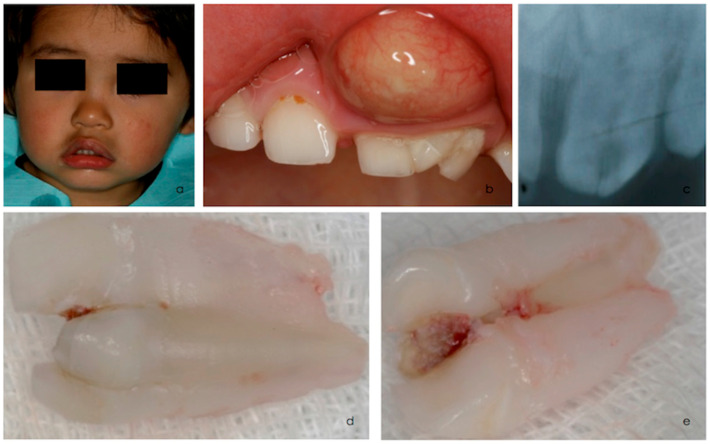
(a–c). Extraoral and intraoral exam. Intraoral periapical radiograph shows fusion of two primary incisors with supernumerary tooth, with separate pulp chamber and root canals. Based on the clinical and radiographic findings, the diagnosis of triple teeth was confirmed. (d,e) The treatment plan was aimed to extract the offending tooth with local anesthesia, under antibiotic prescription.
